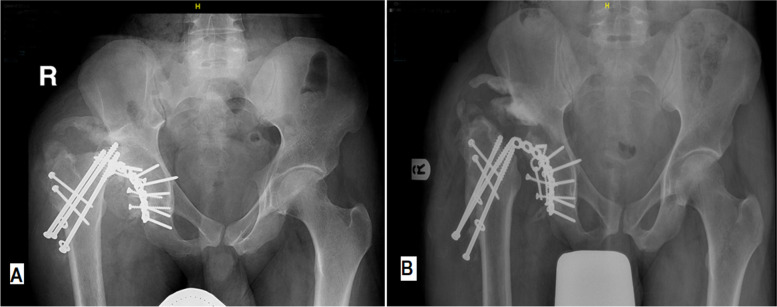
Patient with HO developed treatment failure postoperatively. (X-ray for the patient with Rt hip HO, A showed preoperative HO and B showed 3 months postoperative treatment failure)
